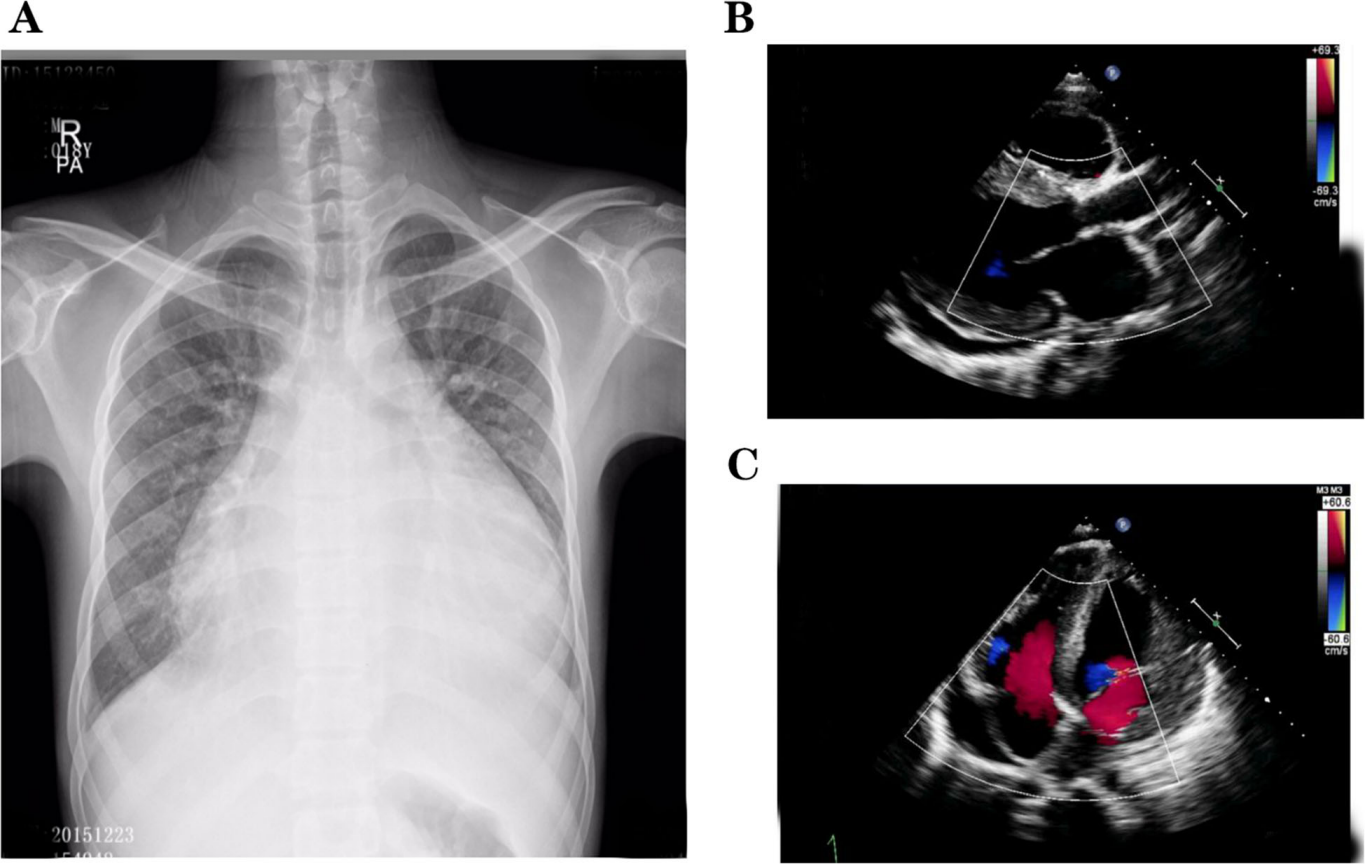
Chest radiographs and transthoracic echocardiography assessment of the proband. a Chest radiographs suggest bilateral enlargement of the heart. b, c Transthoracic echocardiography shows symmetrical hypertrophy, dilatation of all four cavities, and moderate amount of pericardial effusion
